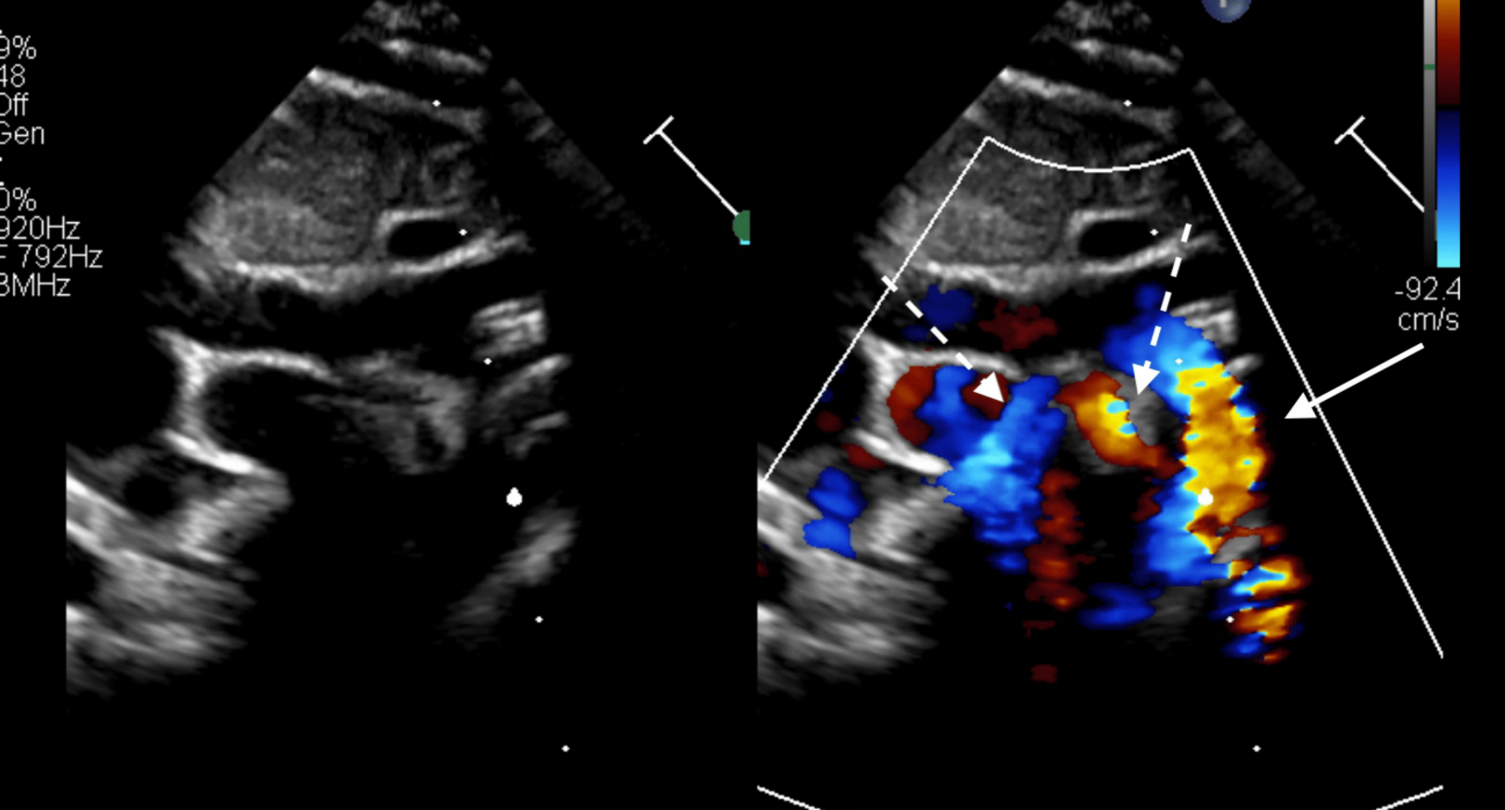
Echocardiography (arch view) Echocardiography showed an abnormal vessel originating from the descending aorta. The solid arrow indicates the aortic arch and the dashed arrow indicates the abnormal vessel.
